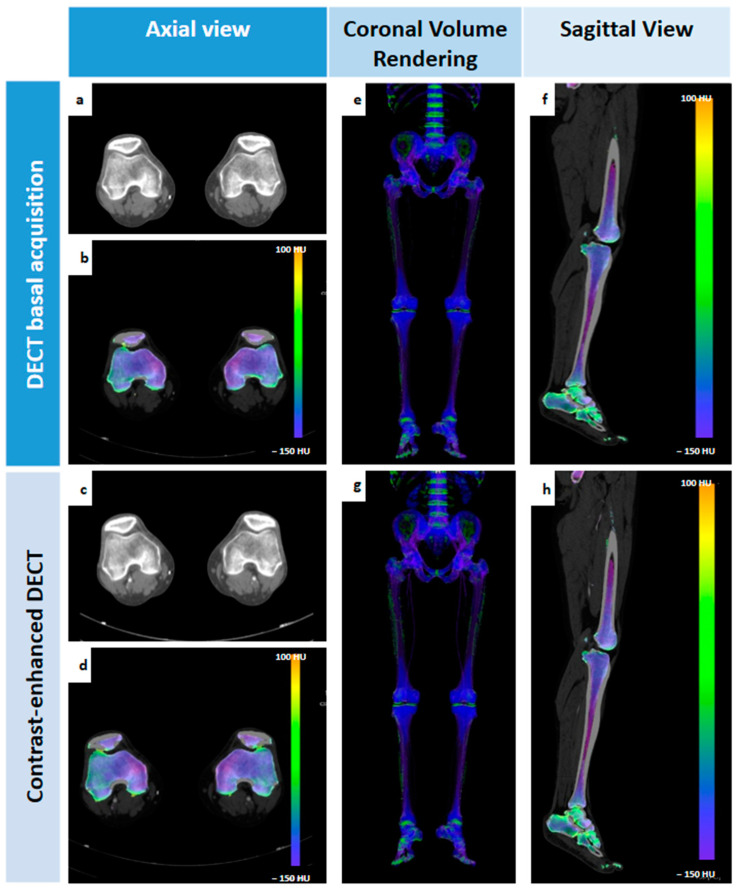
DECT axial unenhanced (a), mixed 120 KvP image of the lower limbs at knee level with its corresponding VNCa image (b), and analogous DE-CTA axial mixed 120 KvP image (c) with its corresponding VNCa image (d), showing no qualitative differences in the colorimetric map of bone marrow attenuation. Again, no visual differences are present between unenhanced (e) and enhanced (g) 3D volume rendering images and between unenhanced (f) and enhanced (h) sagittal reformatted colorimetric maps of bone marrow attenuation.
